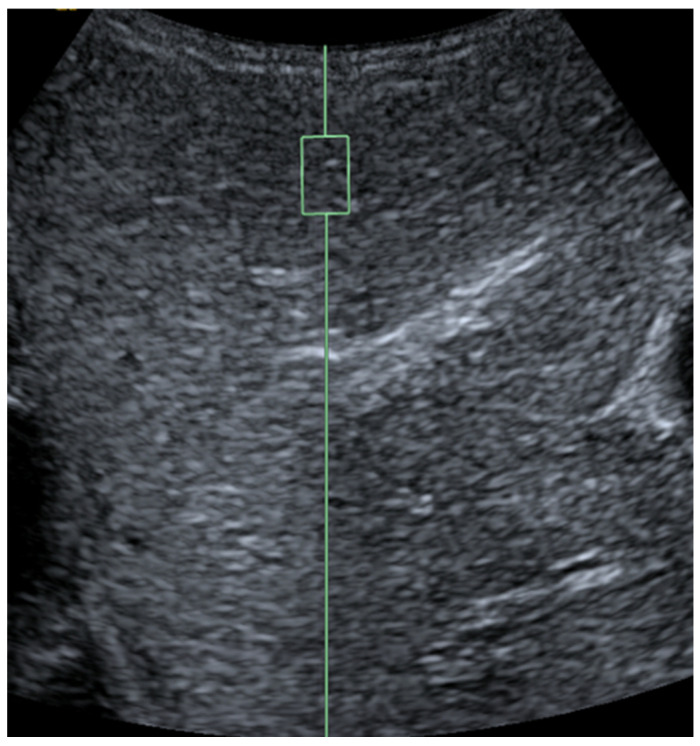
Congenital hepatic fibrosis in a 5-year-old girl. Mean shear wave elastography at the right lobe of the liver measured 3.9 m/s. The normal pediatric liver stiffness value using this ultrasound scanner and technique is approximately 1.16 m/s (SD +/− 0.14 m/s). Therefore, the finding is suggestive of fibrosis.
